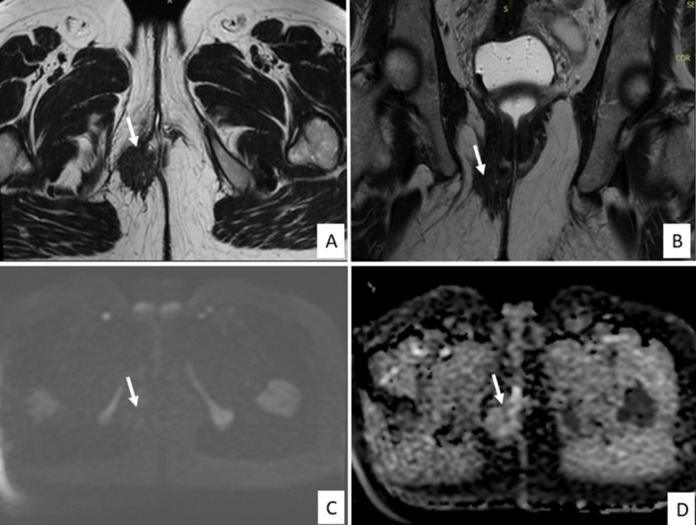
axial (A) and coronal (B) T2-weighted MR images demonstrating a right-sided perineal mass with irregular, stellate contours extending toward the posterolateral wall of the distal third of the vagina and infiltrating the subcutaneous fat; The lesion exhibits low signal intensity on T2-weighted sequences, interspersed with small hyperintense cystic foci; no diffusion restriction is observed on DWI and ADC images (C, D)
